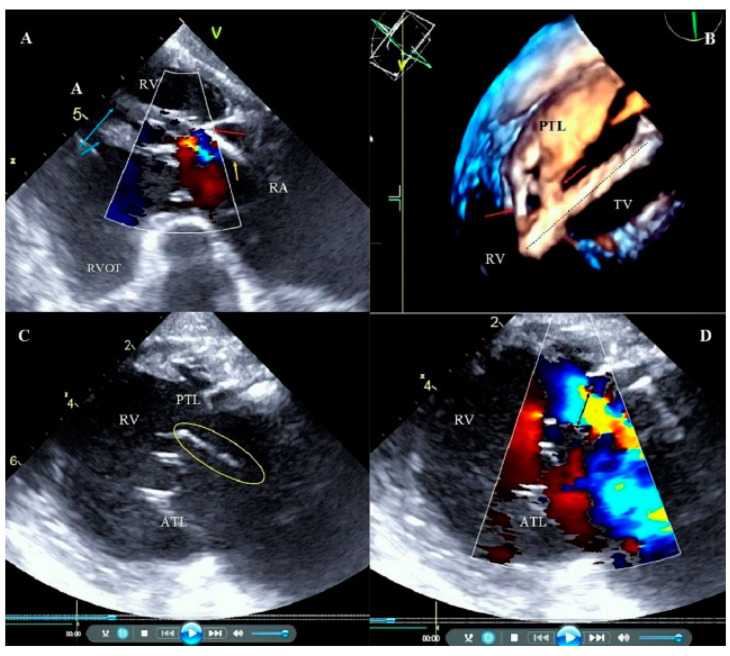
Adhesion of the lead to the tricuspid apparatus. Broken chordae tendineae during transvenous lead extraction (TLE). (A) 2D transesophageal echocardiography (TEE) transgastric view, color Doppler. The ventricular lead (yellow arrow) is fused with the posterior leaflet (red arrow) and the subvalvular apparatus. Papillary muscles (blue arrows). Low tricuspid regurgitation. (B) 3D TEE. Tricuspid valve (TV) view from the right ventricle (RV) side. Tissue bridges (adhesions) (red arrows) connecting the ventricular lead (dashed line) with the posterior leaflet (PTL). (C) 2D TEE transgastric view. Broken chordae tendineae (circle) that moves to right atrium (RA) (D) 2D TEE transgastric view. Image from panel C in the option of color Doppler, large TR to RA, Vena contracta (VC) = 11 mm (black arrow).
